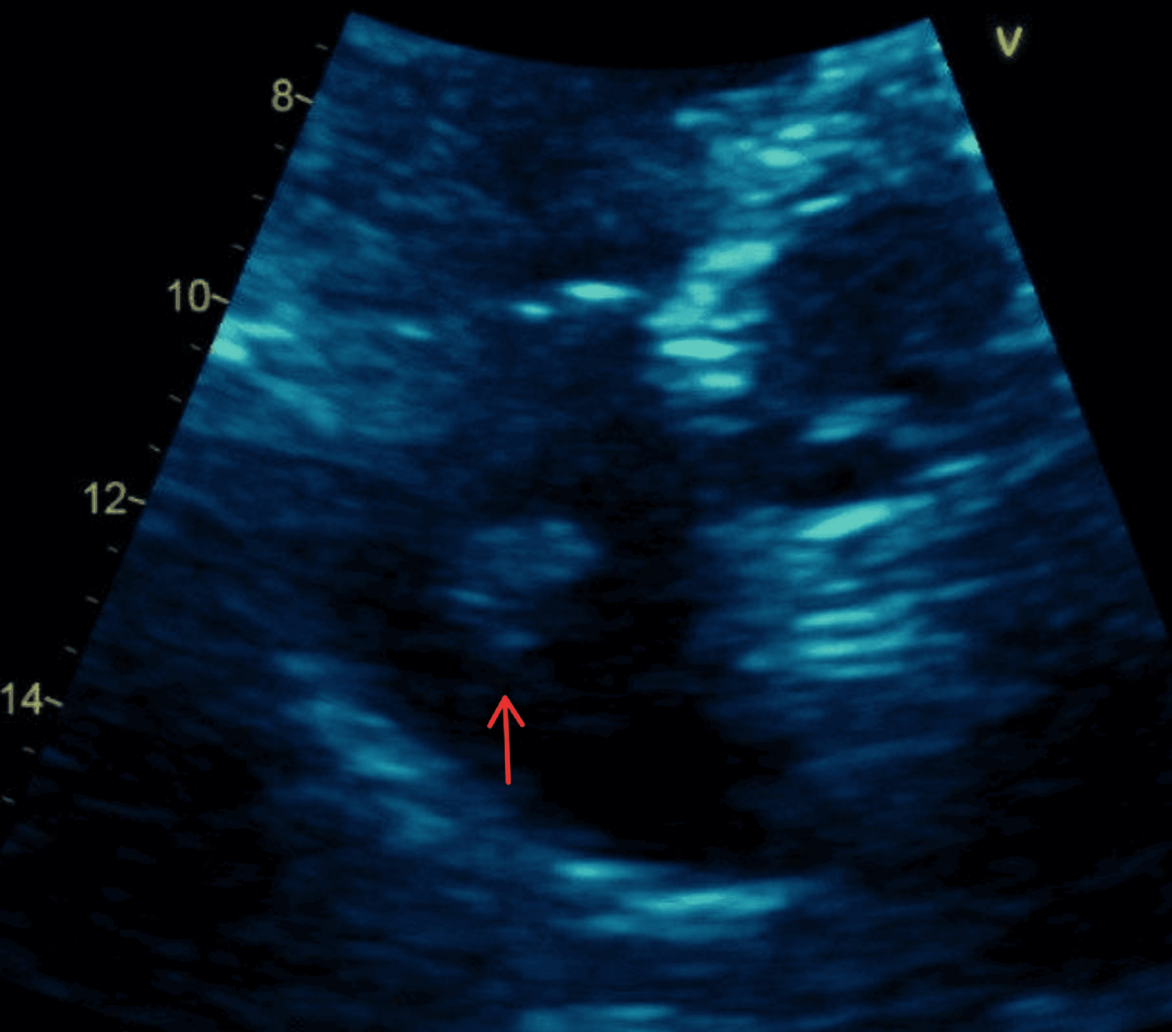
TTE showing the right atrium view with a thrombus in transient (red arrow). TTE: transthoracic echocardiogram
